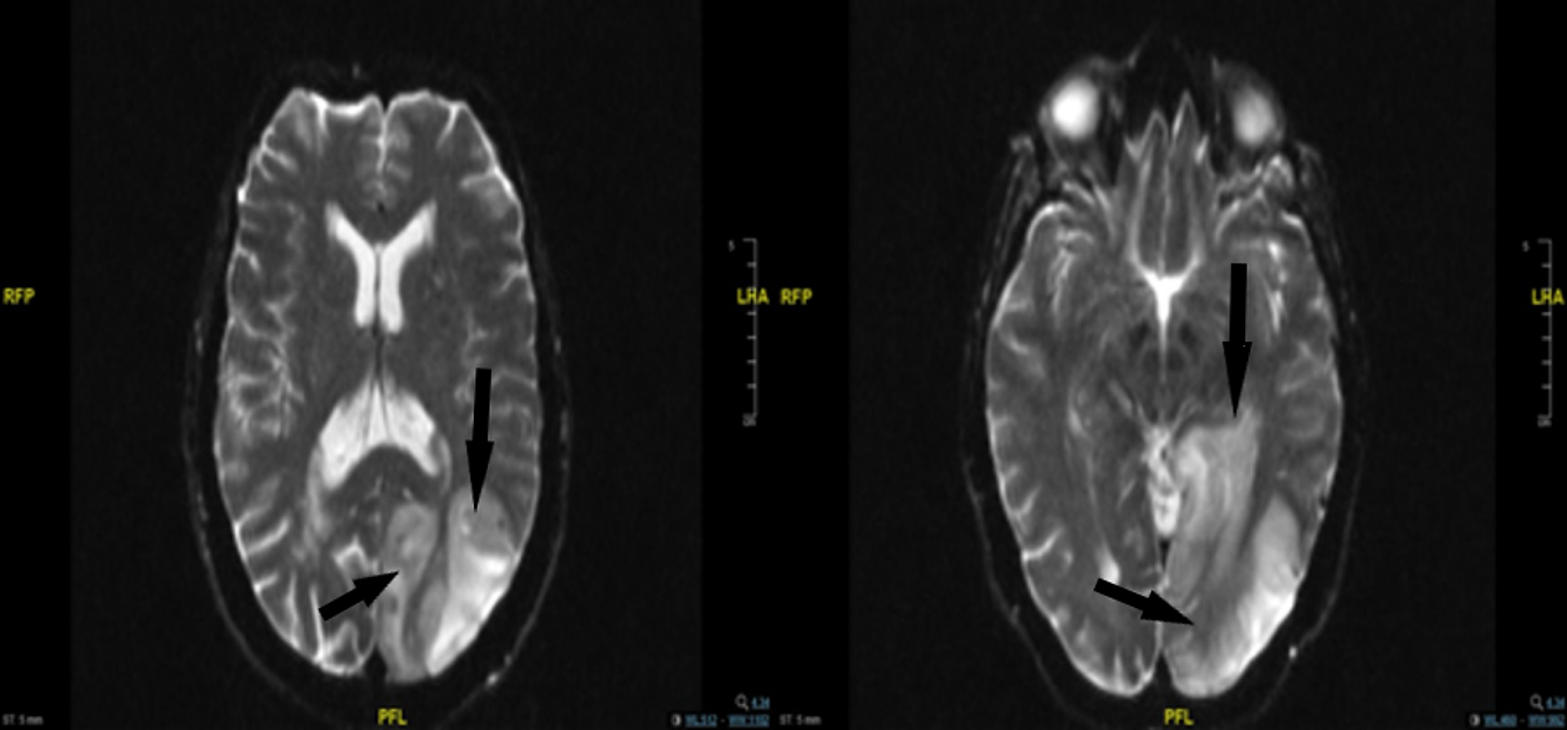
Ep2d diffusion axial MRI head showing a large left occipito-parietal gyriform cortical area of restricted diffusion (black arrows)
